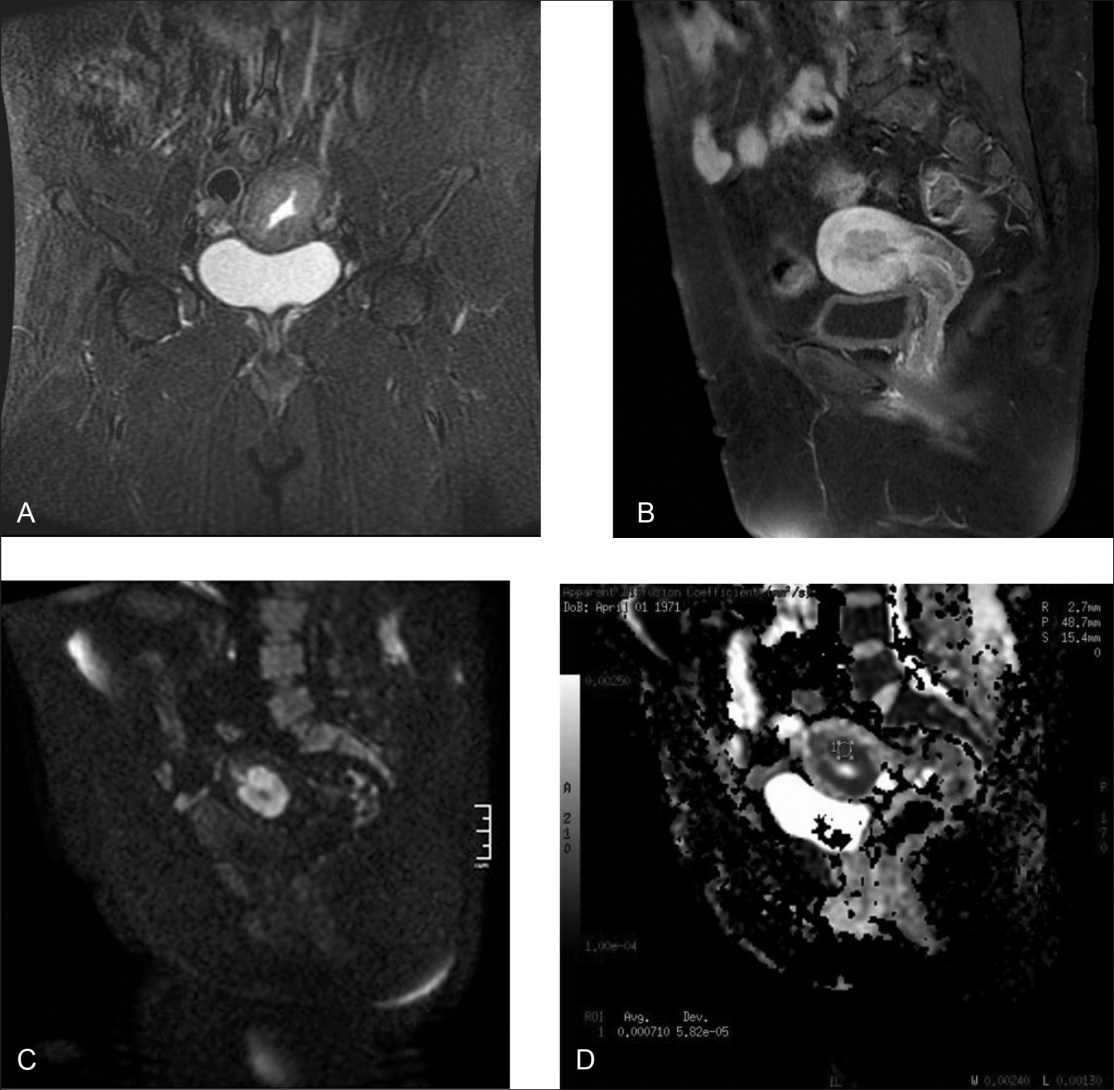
A 42-year-old woman with endometrial cancer. Coronal fast spin-echo T2-weighted image shows the endometrial mass (a). Sagittal gadolinium-enhanced fat-saturated spin-echo T1-weighted image shows the tumor as a slightly enhanced lesion (b). Sagittal diffusion-weighted image (b = 1000s/mm^2^) (c) shows FIGO stage 1B endometrial mass. The ADC value is 0.71x10^–3^ on the sagittal ADC map image (d).
